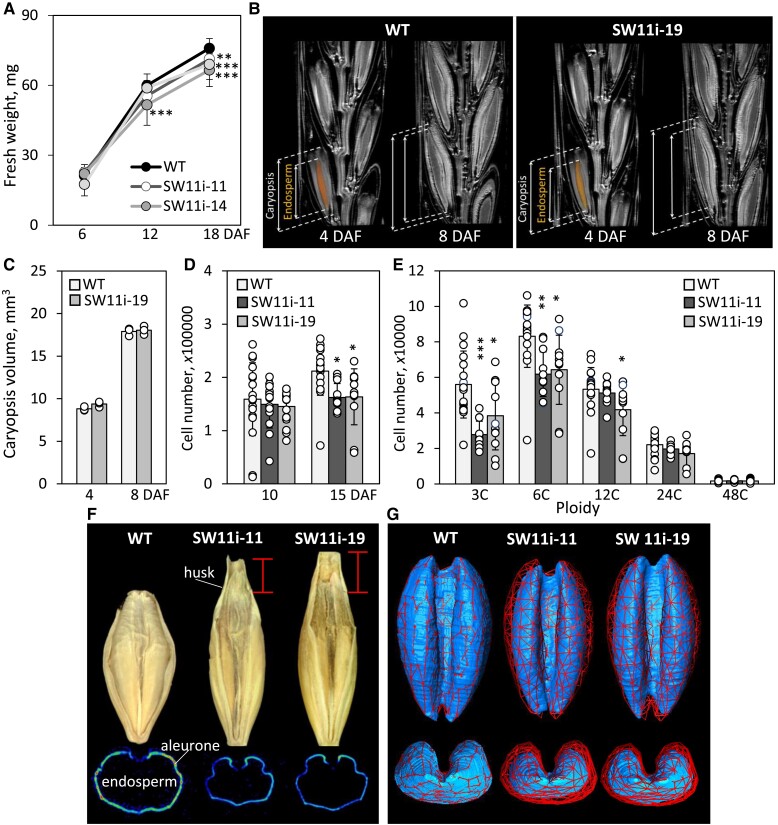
The effect of suppressing *HvSWEET11b* on grain phenotypes. A) Transgenic grains accumulate fresh weight less efficiently than WT. B) Dynamic MRI visualizes grain expansion in WT and *HvSWEET11b*-repressed plants over time (see Supplemental Movie S1). C) The size of developing caryopses at early developmental stages (4 DAF and 8 DAF). D) Cell number in the developing endosperm. E) Ploidy level of endosperm cells at 15 d after flowering. F) The shape of mature WT and *HvSWEET11b*-repressed grains with attached husks (upper panel) and the grain's aleurone layer (lower panel) at virtual cross-sections acquired using MRI. The red lines indicate the greater length of the transgenic grains, resulting from their formation of a longer husk. G) The dorsal (upper panel) and posterior (low panel) aspects of the endosperm in developing grains sampled 12 DAF, as acquired using MRI (see also Supplemental Movie S1). The red network defines the outer surface of the WT endosperm (left). The same network is applied for transgenic endosperms to visualize their similar appearance but smaller size than WT. Bars in (E) and (F): 1 mm. Values are means, error bars represent Sd; *n* = 11 to 42 biological replicates (individual caryopses from at least 6 spikes) in (A), *n* = 3 biological replicates (individual caryopses) in (C), *n* = 12 to 18 biological replicates (individual caryopses from at least 4 spikes) in (D, E). P* < 0.05, *P* < 0.01, and P* < 0.001, as determined by 1-way ANOVA with Bonferroni correction test between WT and the corresponding transgenic line. Individual samples are shown as dots in (C–E). DAF, days after flowering.
